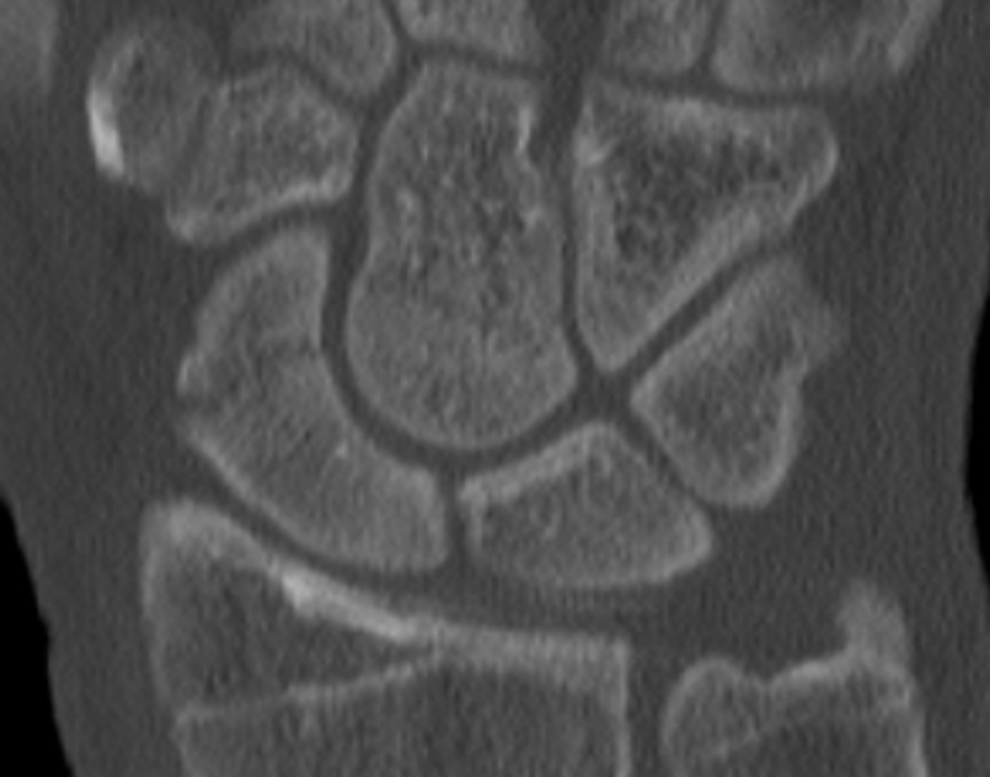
CT image of scaphoid and capitate fracture in patient no. 16
